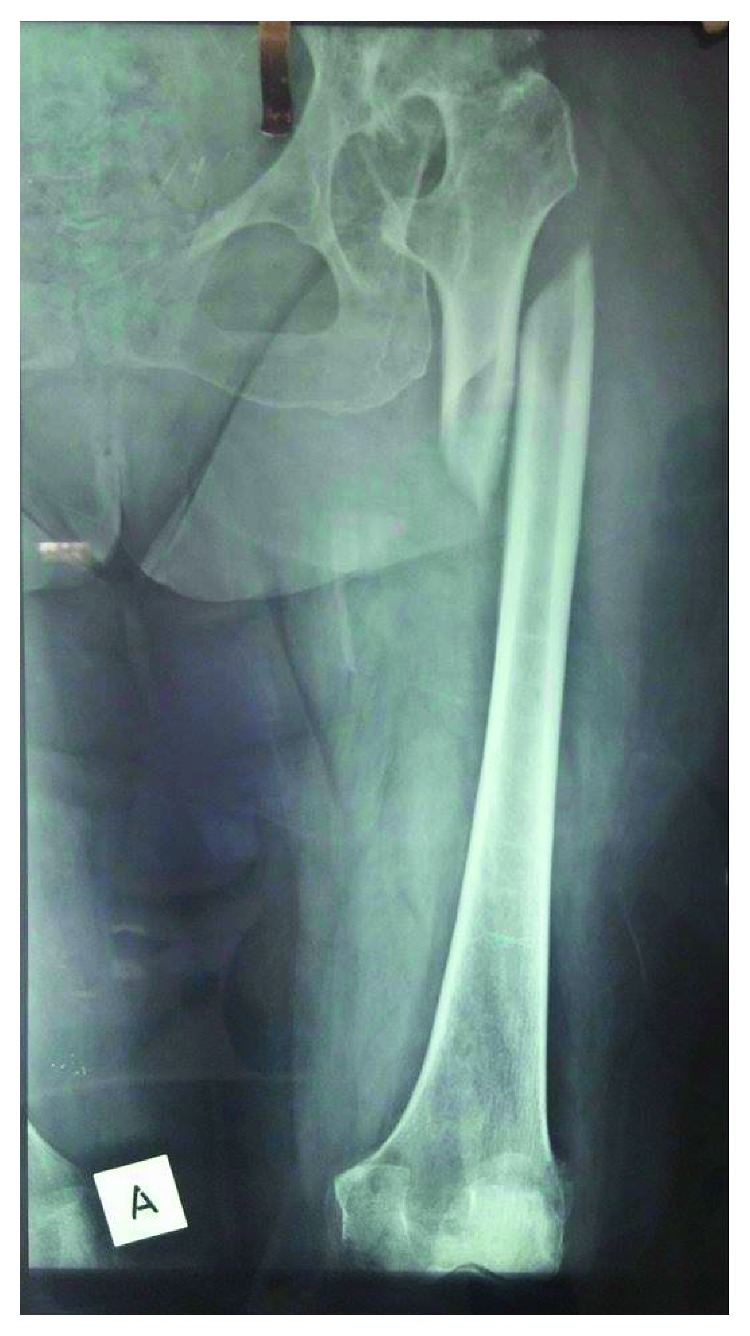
Initial posttraumatic anteroposterior radiograph of the left femur. Congenital hip disease and spiral shaft fracture of the proximal third of the femur are revealed.
